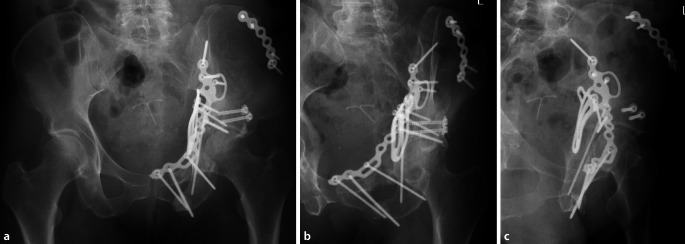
Die Analyse der Schraubenlage wurde mit der Open-Source-Software OsiriX Lite (Fa. Pixmeo, Bernex, Schweiz) durchgeführt. Die postoperativen Computertomographie(CT)-Scans aller Patienten wurden multiplanar 3D-rekonstruiert. Der IAC wurde nach der von Egli und Kanezaki beschriebenen Technik identifiziert [12, 13]. Der infraazetabuläre Diameter (IAD) wurde als die schmalste Stelle des Korridors auf der Inlet-Aufnahme des Beckens in kaudaler Inklination definiert (Abb. 3). Die Schrauben-Korridor-Distanz (SCD) wurde auf der axialen Aufnahme als kürzeste Distanz zwischen dem Zentrum des IAC und dem Zentrum der Schraube festgelegt (Abb. 4). Der Schrauben-Knorpel-Abstand (SFCD) wurde nach Darstellung der gesamten Schraubenlänge im CT als Abstand des Schraubenkopfes zum Femurkopfknorpel definiert (Abb. 5). Der Schrauben-Korridor-Winkel (SCA) wurde definiert als der Winkel zwischen der Achse, die durch das Zentrum der Schraube verläuft, und der Achse, die durch das Zentrum des IAC verläuft. Beide Achsen haben ihren Ursprung an der Eintrittsstelle der Schraube in den Knochen (Abb. 6).
